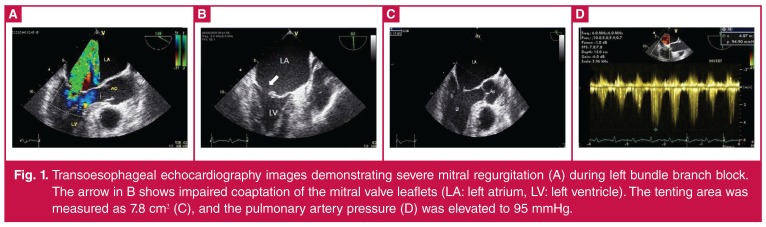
Transoesophageal echocardiography images demonstrating severe mitral regurgitation (A) during left bundle branch block. The arrow in B shows impaired coaptation of the mitral valve leaflets (LA: left atrium, LV: left ventricle). The tenting area was measured as 7.8 cm2 (C), and the pulmonary artery pressure (D) was elevated to 95 mmHg.
